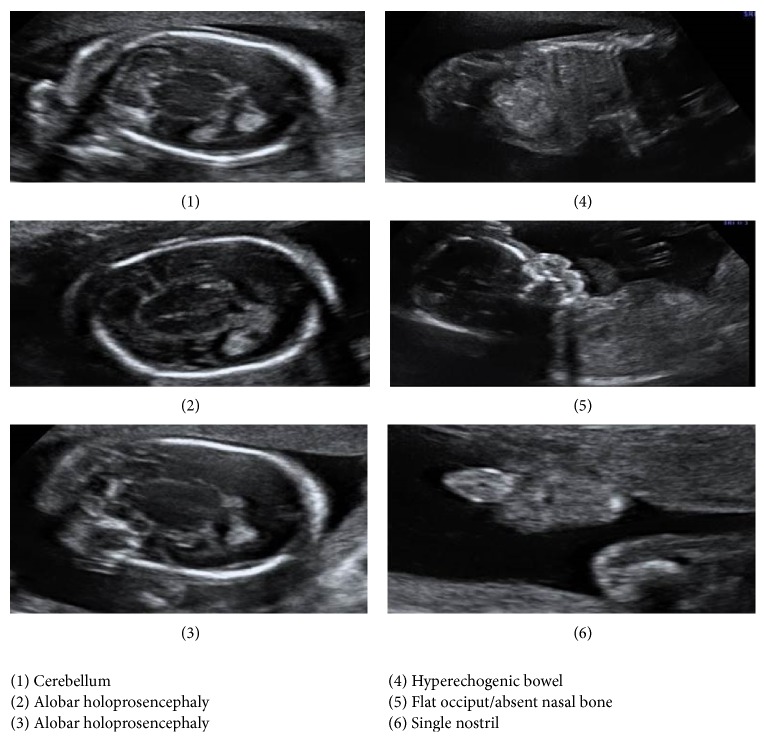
Ultrasound images of the anomaly scan performed at 20 weeks and 3 days.
